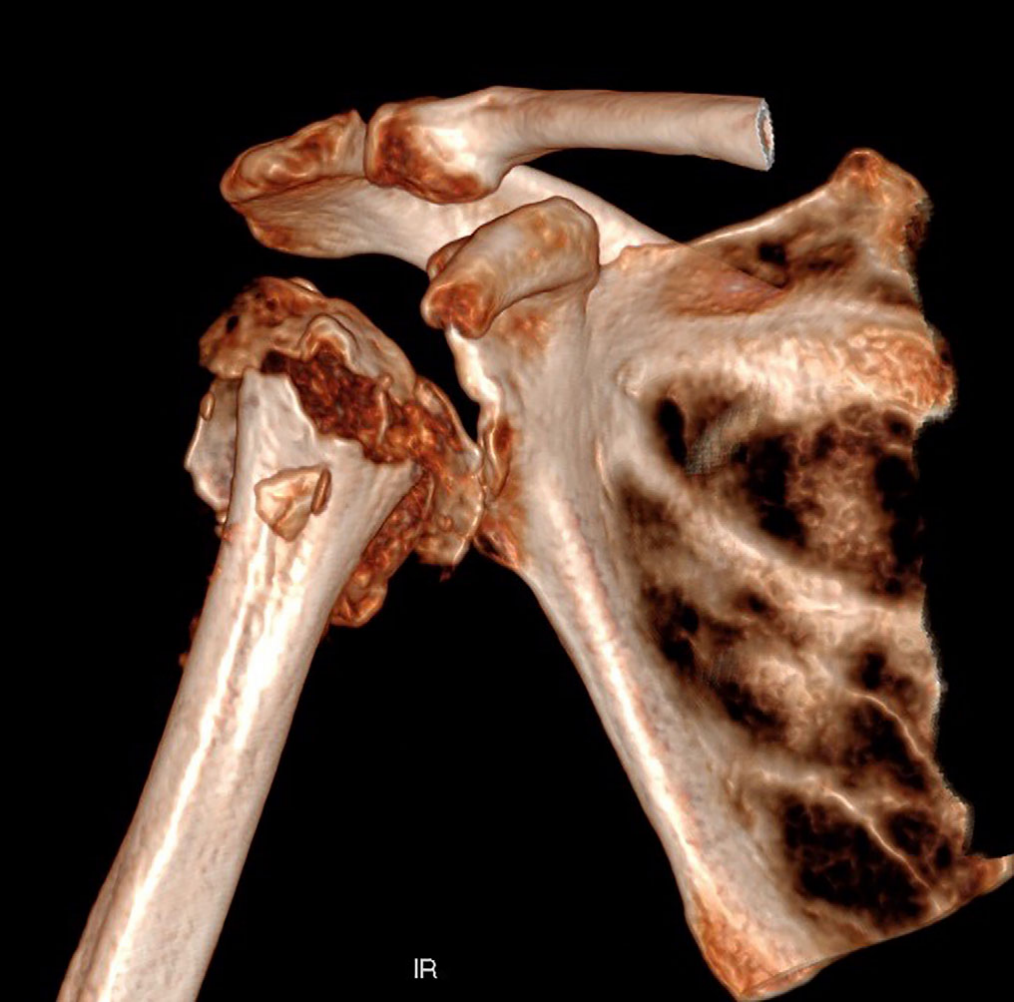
Three‐dimensional CT image displaying a four‐part fracture with humeral head displacement from the shaft. The dent in the glenoid is visible. Head split component with small medial head piece in varus configuration.
